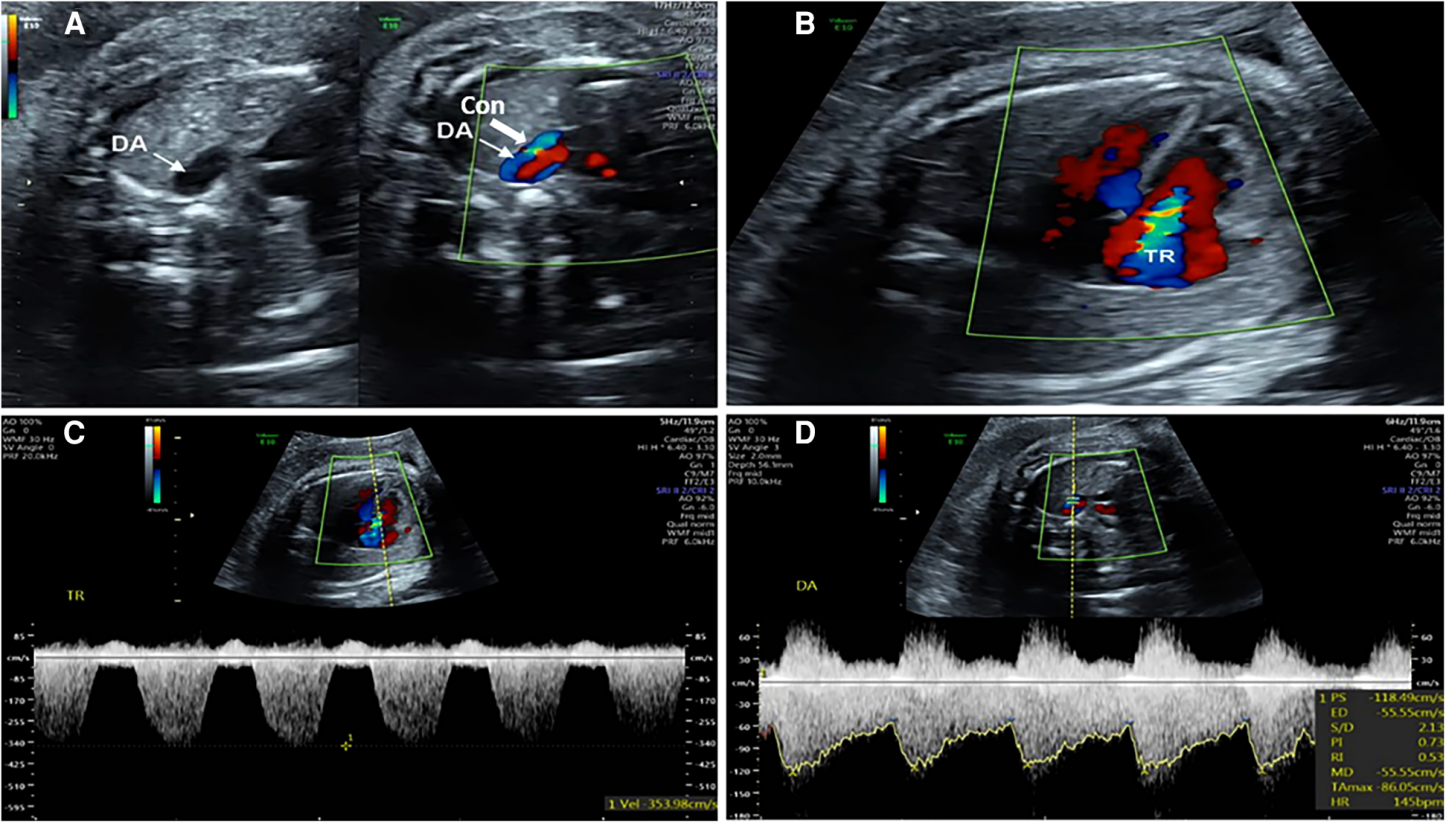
Echocardiograms of the fetuses with ductus arteriosus premature contraction (DA Con). (A) Narrowed ductus arteriosus; (B) Full-systolic tricuspid regurgitation (TR) on four-chamber view; (C) Velocity of TR; (D) Blood flow velocity and pulsatility index (PI) value of DA Con.
